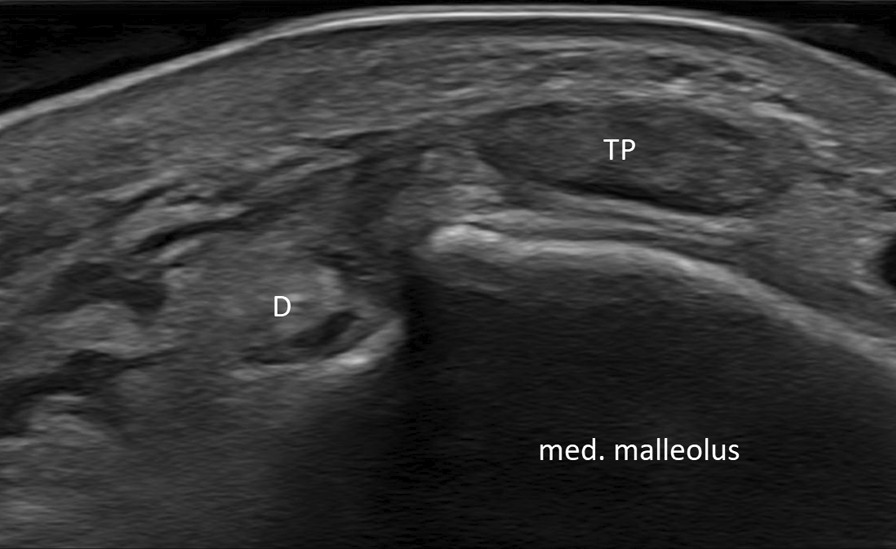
Ultrasound of the left ankle showing the posterior tibial tendon (TP) on top of the medial malleolus. *D* flexor digitorum tendon
